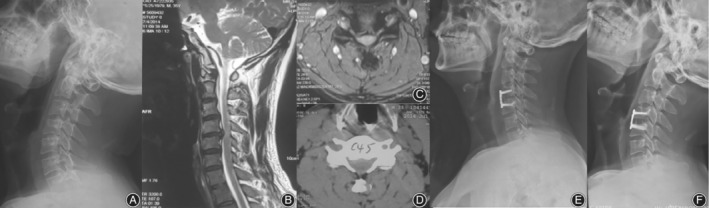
A 35‐year‐old male patient with proximal‐type CSA at the C_4/5_ level. (A) The pre‐operative X‐rays of the cervical spine showing narrowed intervertebral space of the C_4/5_. (B) Sagittal T2‐weighted magnetic resonance image showing cord compression at the C_4_‐C_5_ space; (C) Axial T2‐weighted magnetic resonance image at the C_4_‐C_5_ space showing impingement against the right ventral nerve root and anterior horn, with no abnormal signal intensity in the spinal cord. (D) CT of the cervical spine show that the center of the C_4/5_ disc protrudes to the right, the outlet of the right nerve root is narrowed. (E) postoperative lateral X‐ray of the cervical spine showing that the internal fixation is firm. (F) Follow‐up at 3 months, X‐ray of the cervical spine showing that the internal fixation is firm and the bony fusion is formed.
